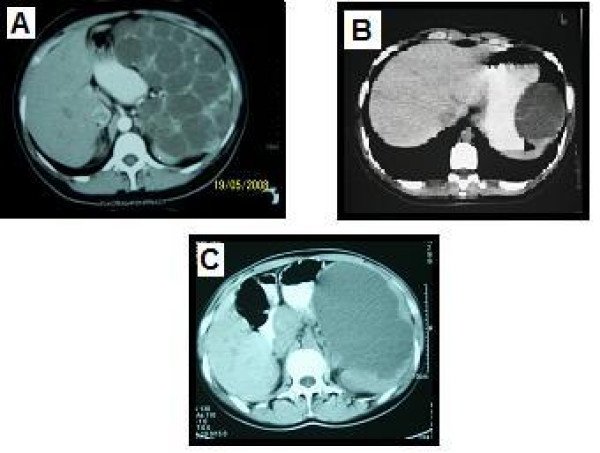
CT view of the different cyst. A) Cystic lenfangiomas B) Epidermoid cyst C) Cyst hidatic.
